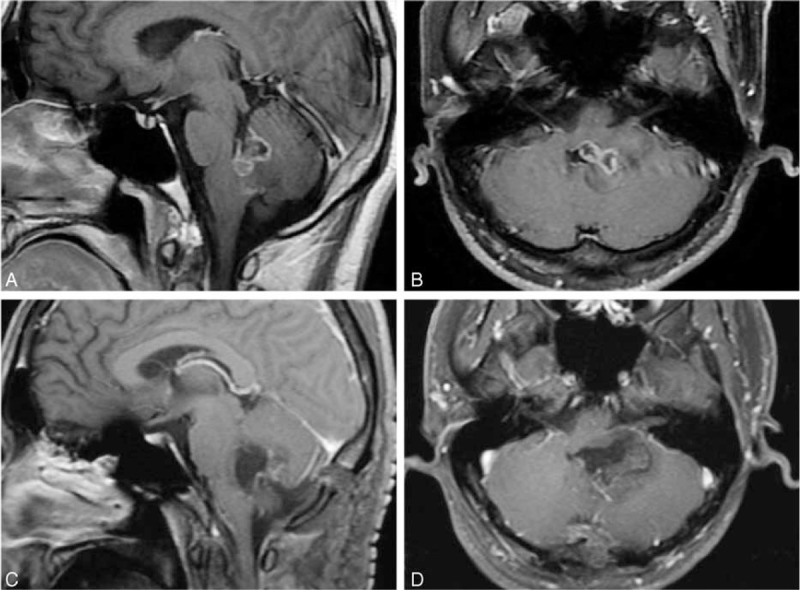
Preoperative sagittal (A) and axial (B) Gd-enhanced T1-weighted MR images demonstrating a heterogeneous ring-enhancement lesion involving left brachium pontis region and extending into the 4th ventricle. Sagittal (C) and axial (D) Gd-enhanced T1-weighted MR images obtained on the second day after surgery evaluation with no evidence of tumor. Gd = gadolinium, MR = magnetic resonance.
